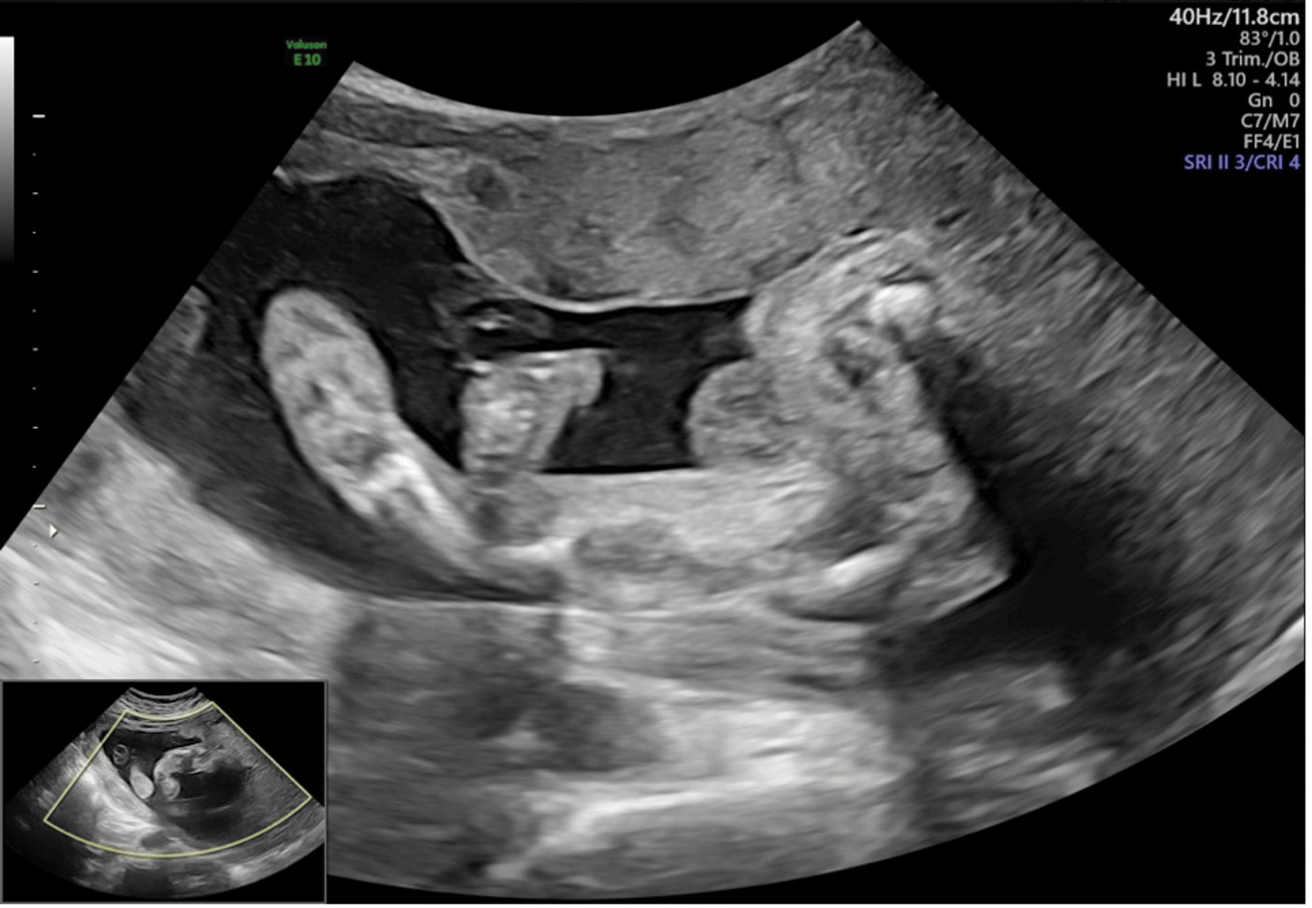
Antenatal ultrasound. Antenatal ultrasound demonstrates multiple structural anomalies, notably involving the femurs and feet, including clubfoot deformities.
